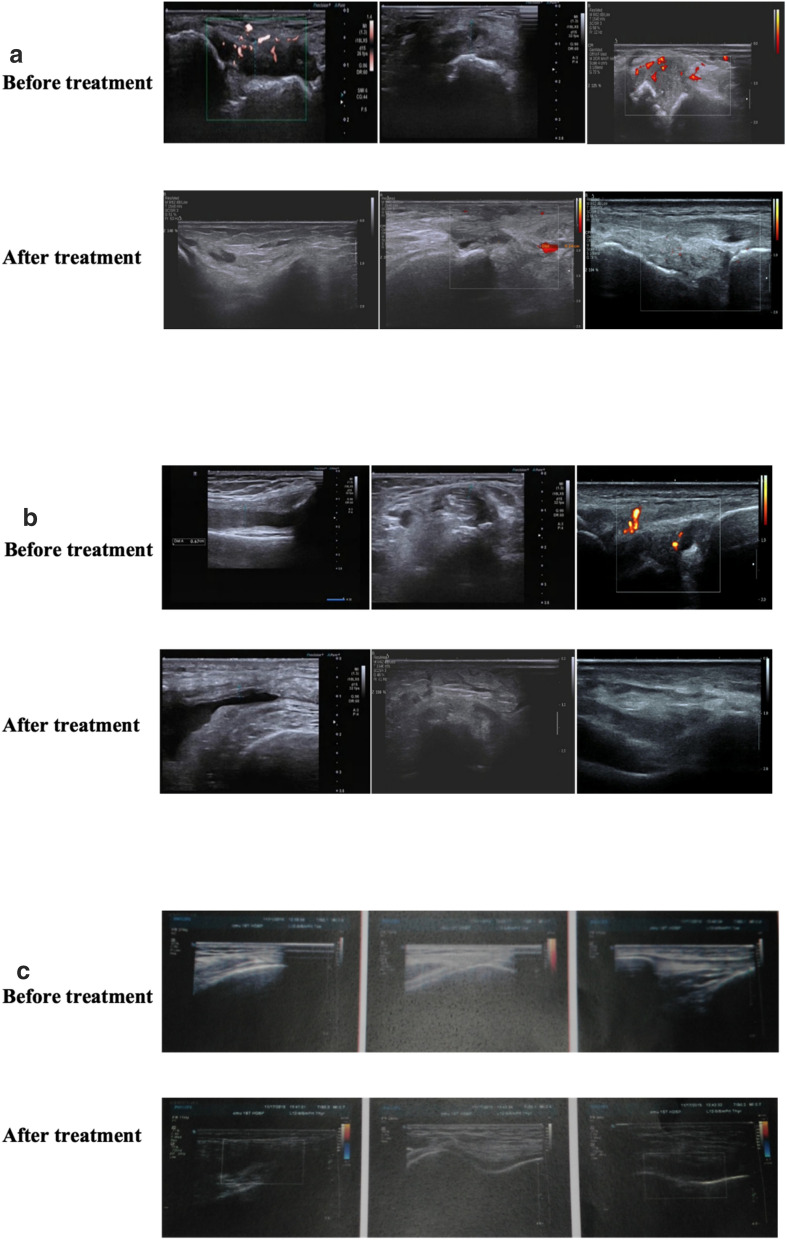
The measurement of synovial thickness of joint under color ultrasound in Control group (a), T1 group (b), T2 group (c)
